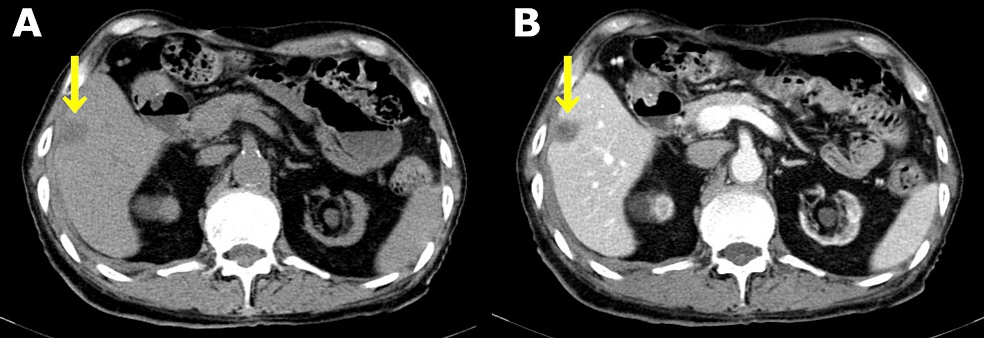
Follow-up CT findings in the liver A: The hepatic SOL in plain CT (yellow arrow). B: The hepatic SOL with a slightly high density in the arterial phase (yellow arrow) CT: computed tomography; SOL: space-occupying lesion
